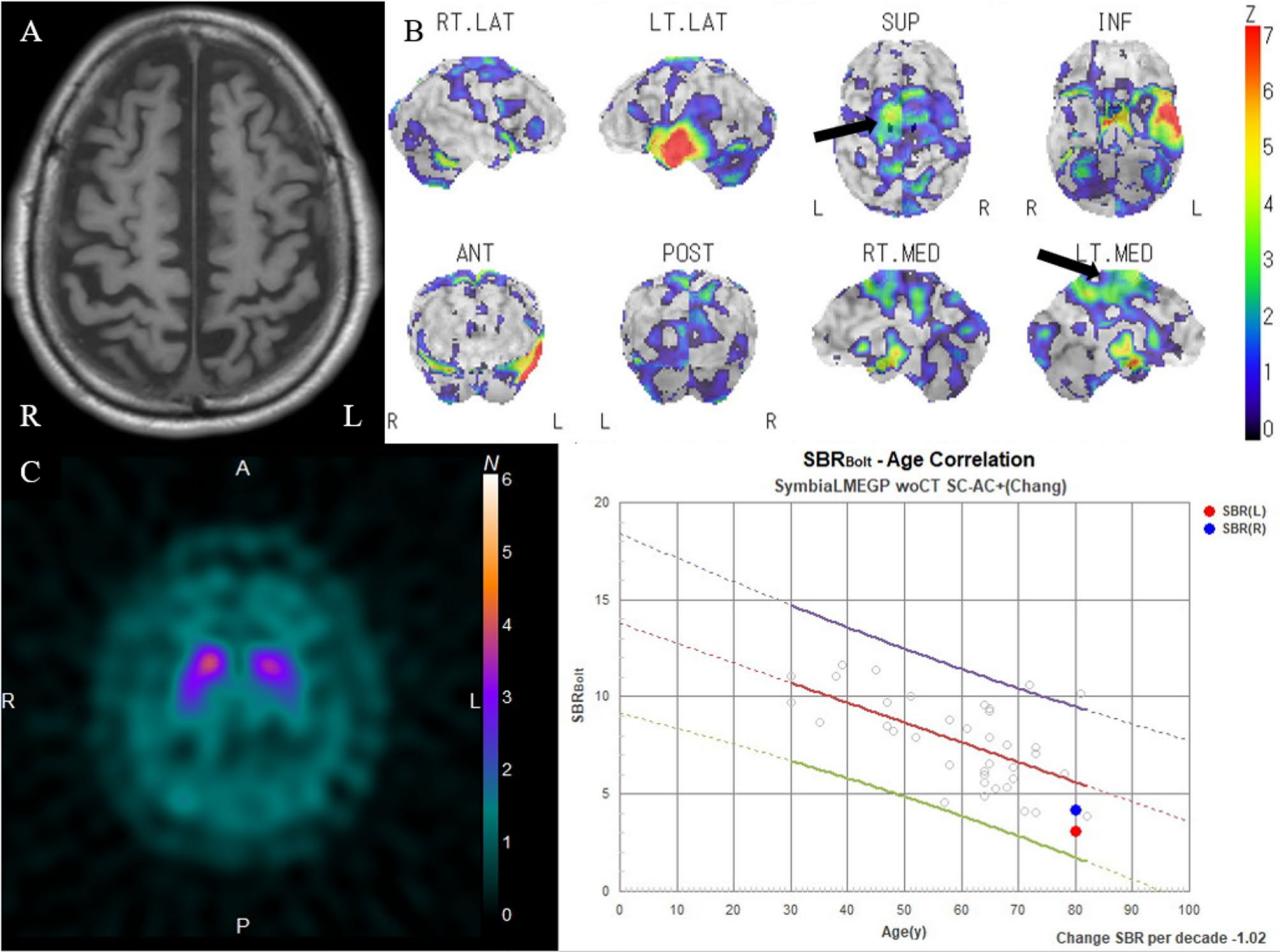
Magnetic resonance images (MRIs) and three-dimensional stereotactic surface projection (3D-SSP) analysis images of 123I-IMP SPECT of case 2. Axial T1-weighted image shows atrophy of the left temporal lobe due to prior cerebral infarction and generalized atrophy, particularly in the parietal lobes (A). 3D-SSP analysis images of 123I-IMP SPECT indicate a reduction in regional cerebral blood flow in the left dorsal SMA (arrow), without basal ganglia reduction. Color-coding represents the statistical significance (Z-score) of the decrease in regional cerebral blood flow (B). A dopamine transporter scan demonstrates visually mild left-dominant hypo-accumulation, with specific binding ratio values of 4.17 and 3.06 on the right and left sides, respectively (C)
